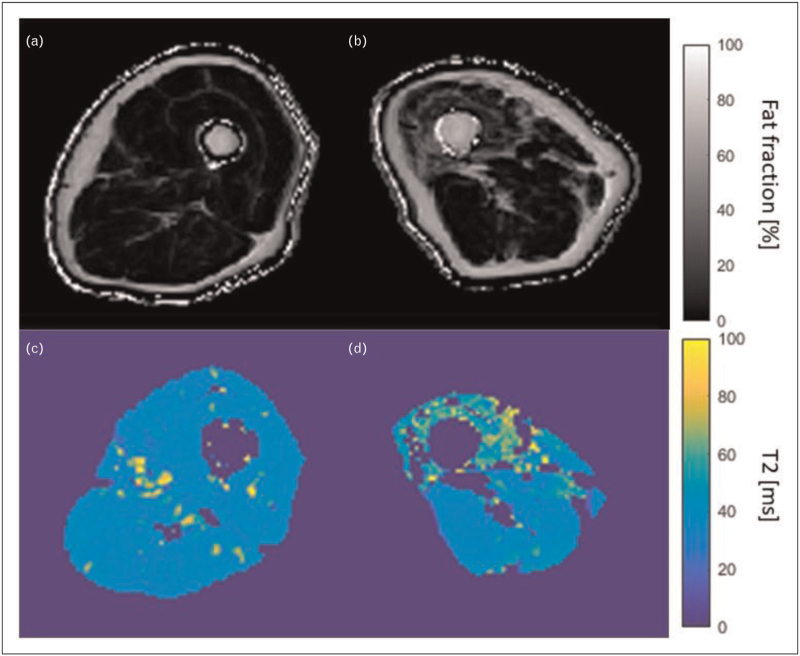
Example of MRI fat fraction (a and b) and T2 maps (c and d) of the thigh muscles in a myositis patient (b and d) as compared to those in a healthy volunteer of similar age and the same sex (a and c). Raised fat content and increased T2 in the quadriceps are indicative of myositis in the patient images.
